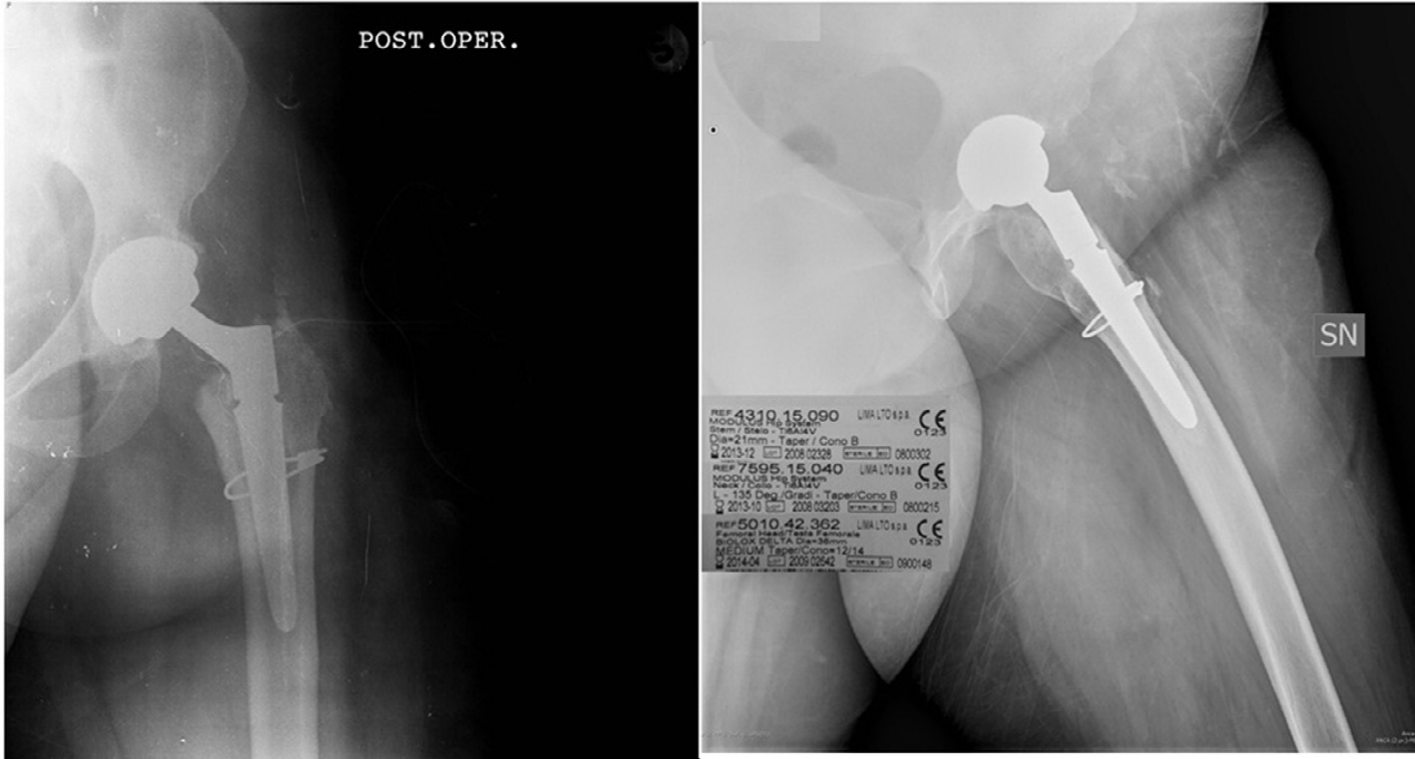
Postoperative X-ray after the Metha stem revision. The 1st implant revision was performed with Lima Modulus implant. Implant details: modulus stem 21 mm diameter, neck Ti6Al4V 135° taper B, Femoral Head 36 mm Biolox delta.
